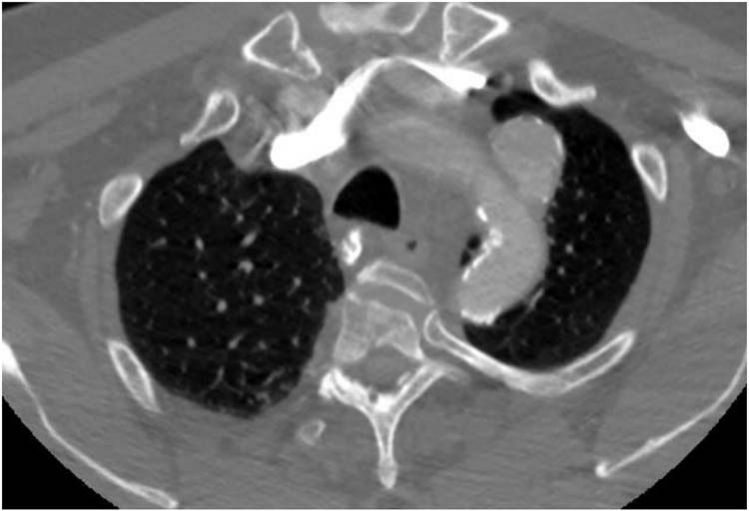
Preoperative CTA demonstrating LSA aneurysm; located at the origin from the aortic arch.
